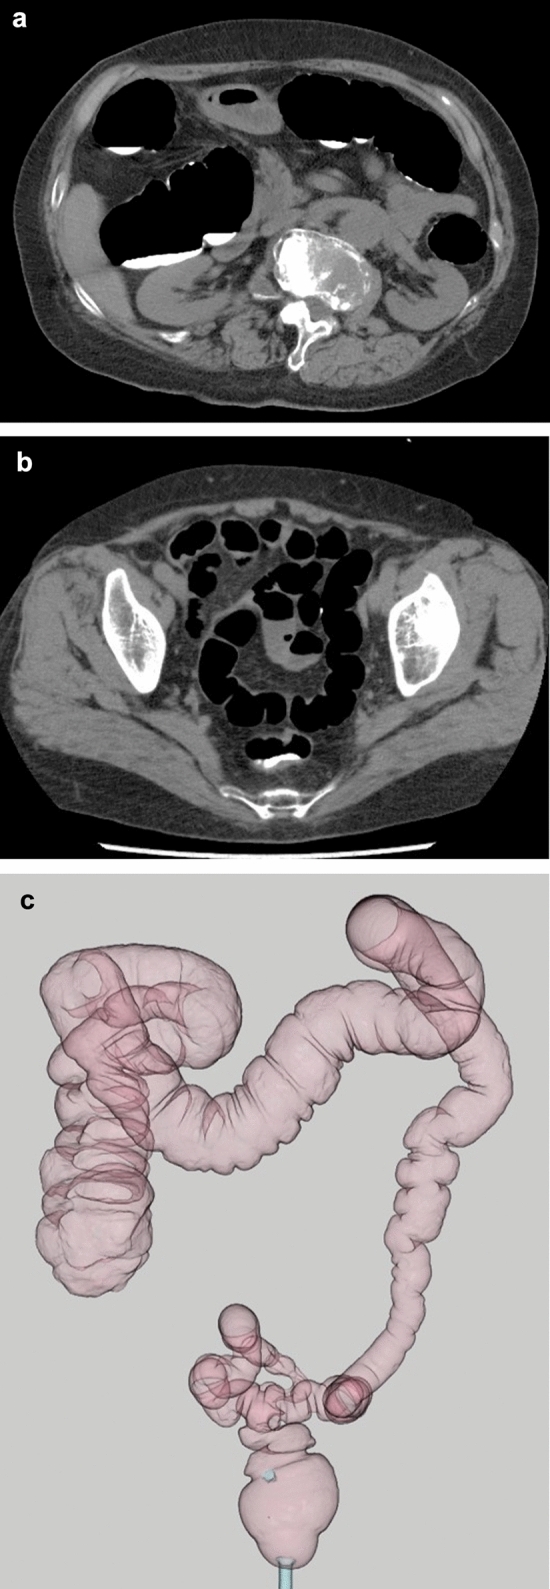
CT colonography in an 81-year-old woman undergoing evaluation for a positive fecal occult blood test. Supine position. A, B Axial CT images showing sufficient luminal distension, minimal residual fluid, and uniform tagging throughout the colon (score 0). In rectum (B), bowel distension is suboptimal (score 1) (arrow). C Barium enema-like view demonstrates generally good distension throughout the colon except for the upper rectum (arrow)
